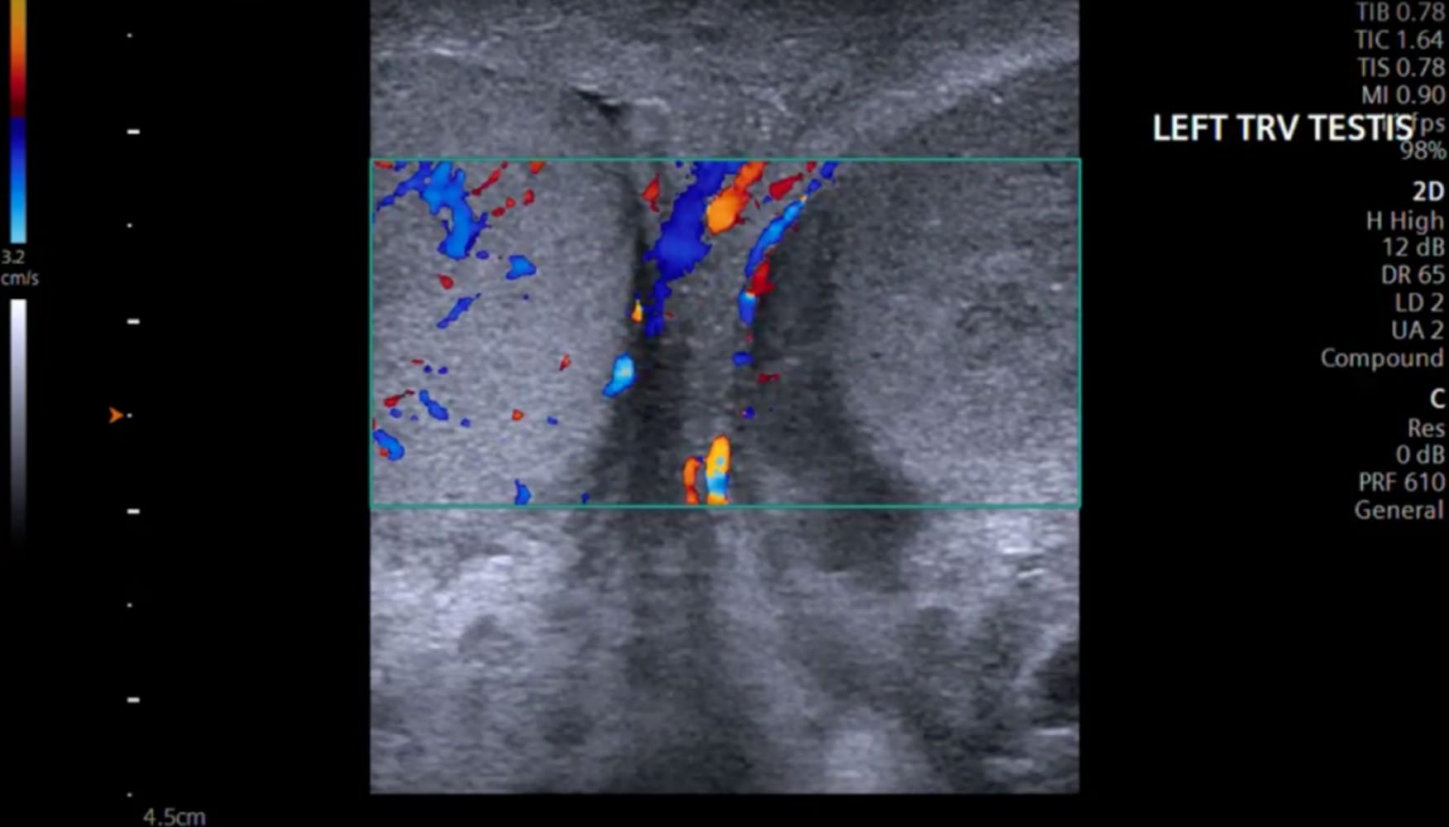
A midline transverse image, including a portion of each testis, is essential in the comparison of echotexture and vascular flow. Here, the right testicle shows asymmetric flow in relation to the left testicle, using Color Doppler with a velocity scale in the order of 3.2 cm/s
